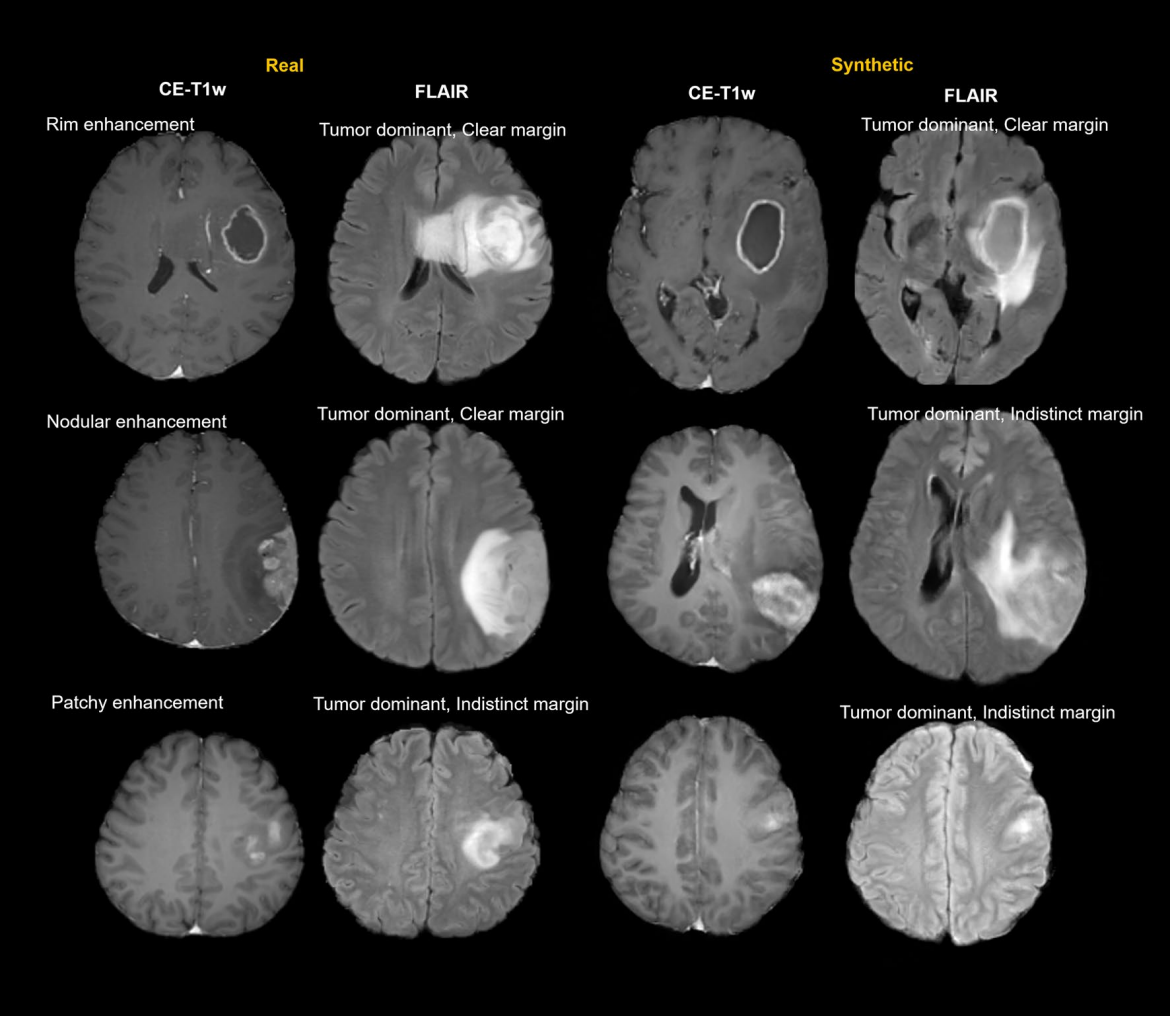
Morphologic characteristics of real IDH-mutant glioblastomas (left) and synthetic IDH-mutant glioblastomas generated by a generative adversarial network (right) based on contrast-enhanced T1-weighted (CE-T1w) and paired FLAIR images. (A) CE-T1w images showing different contrast patterns of rim enhancement, thick nodular enhancement, and patch enhancement. (B) FLAIR images showing types of surrounding high signal intensity (tumor dominant and edema dominant) and margins of non-enhancing lesions (clear and indistinct). Although the appearances of synthetic images are similar to those of real images, there were no exact matches.
